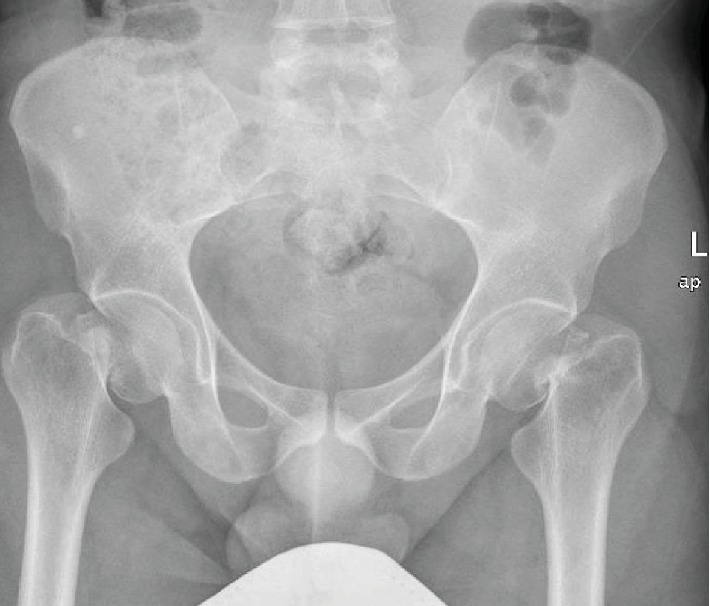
Anteroposterior pelvis radiograph showing the bilateral Garden 4 neck of femur fractures.
